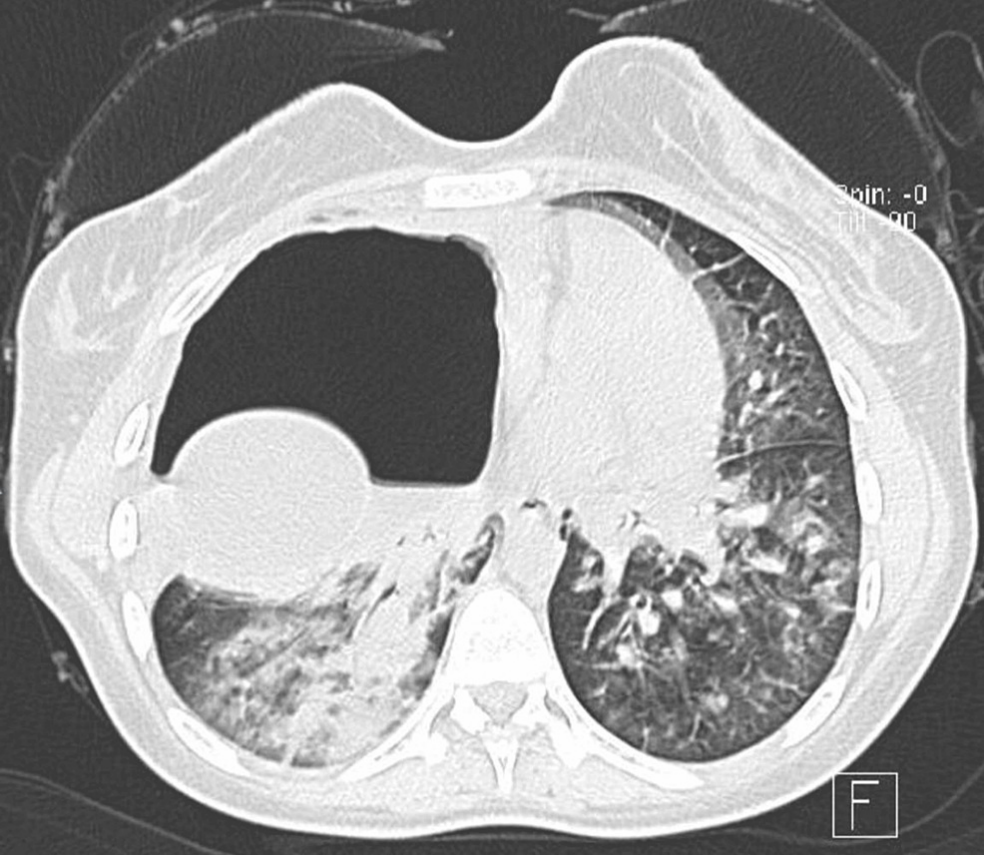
CT chest with contrast, axial plane: bilateral ground glass opacity with a ruptured hydatid cyst in the right lung.
